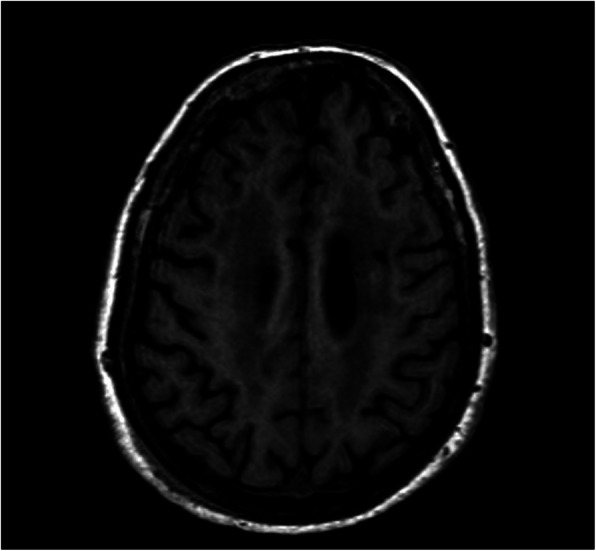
Magnetic resonance imaging revealed a diffuse periventricular (deep and cerebellar) white matter alteration
